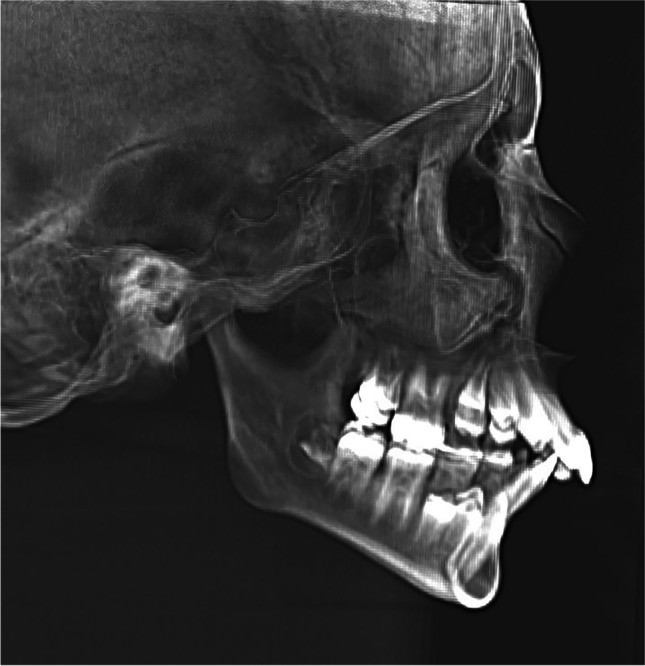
Reconstructed lateral cephalogram from ultra low dose-low dose CBCT
